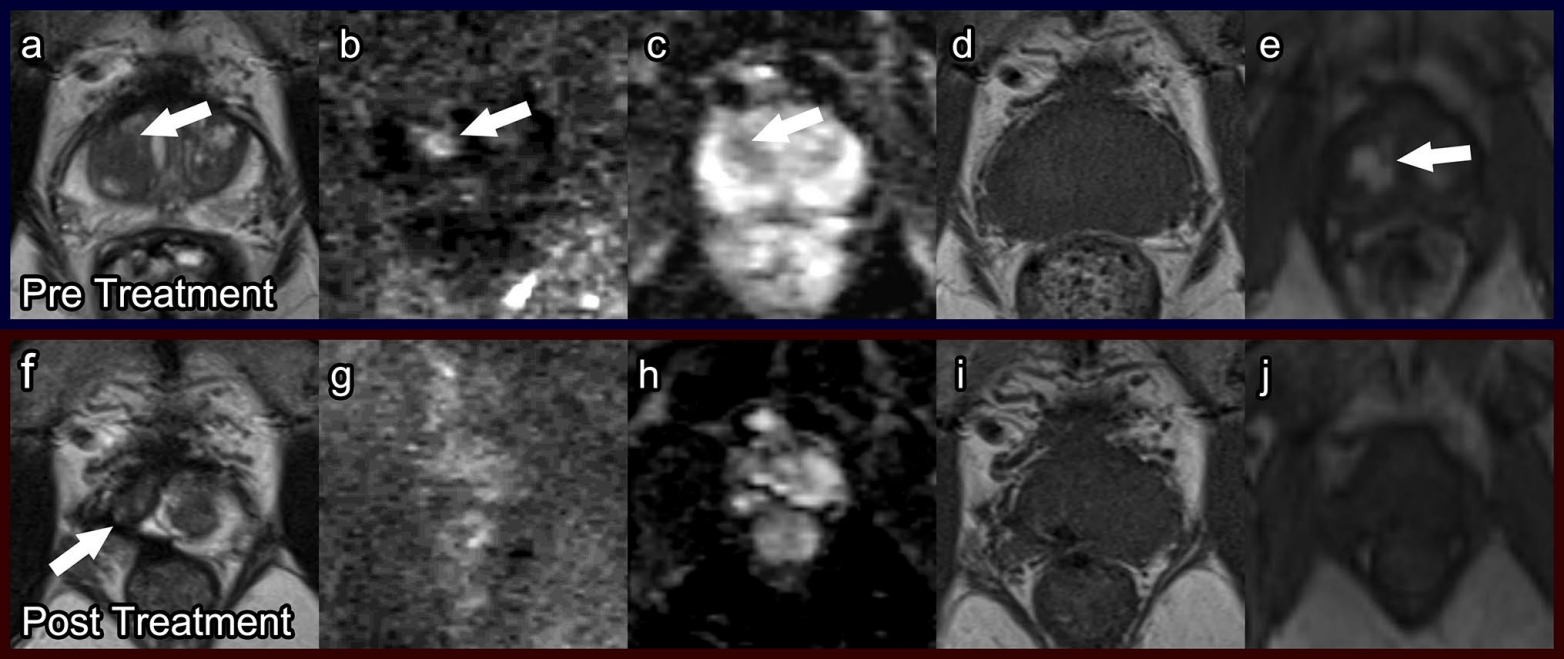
High-intensity focused ultrasound. Pre-treatment prostate MRI: Axial T2 (a), DWI (b), ADC (c), pre-contrast T1 (d), and post-contrast T1 (e) demonstrate a PI-RADS 5 lesion (arrows) in the right mid-transition zone. Biopsied as Gleason score 4 + 3 prostate cancer. Post-HIFU prostate MRI at 1 year: Axial T2 (f), DWI (g), ADC (h), pre-contrast T1 (i), and post-contrast T1 (j) demonstrate linear T2 dark scarring (arrow) and prostatic atrophy without restricted diffusion or hyperenhancement
